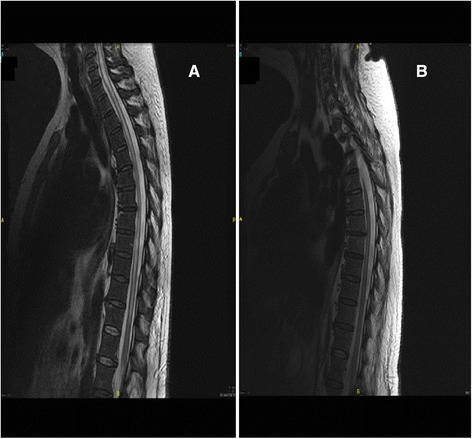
Comparison between the first MRI (figure A) and 2 months late (figure B).
